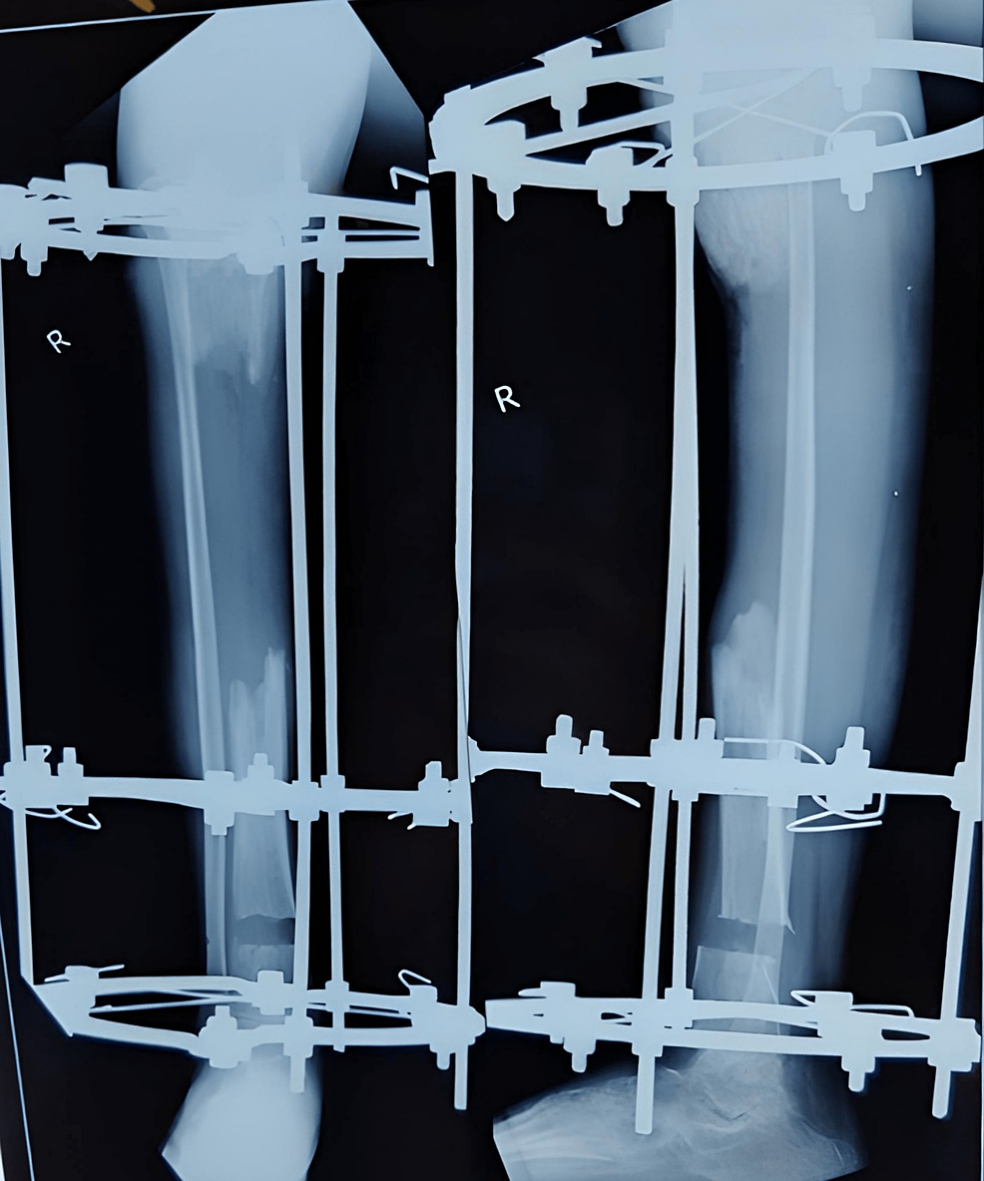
X-ray after corticotomy and Ilizarov application.
